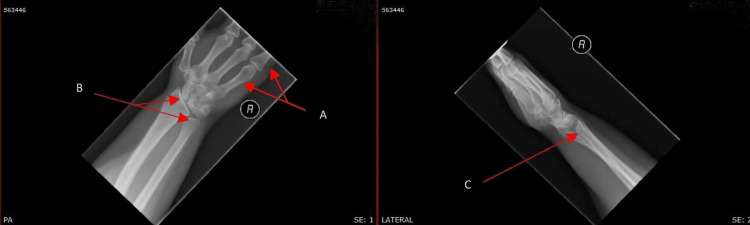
X-ray of the right wrist joint showing absent little finger metacarpal and proximal phalanx (arrow A). Distal radius and ulnar head are slightly dysplastic (arrow B). Diastasis at the distal radio-ulnar joint with negative ulnar variance was noted (arrow C).
